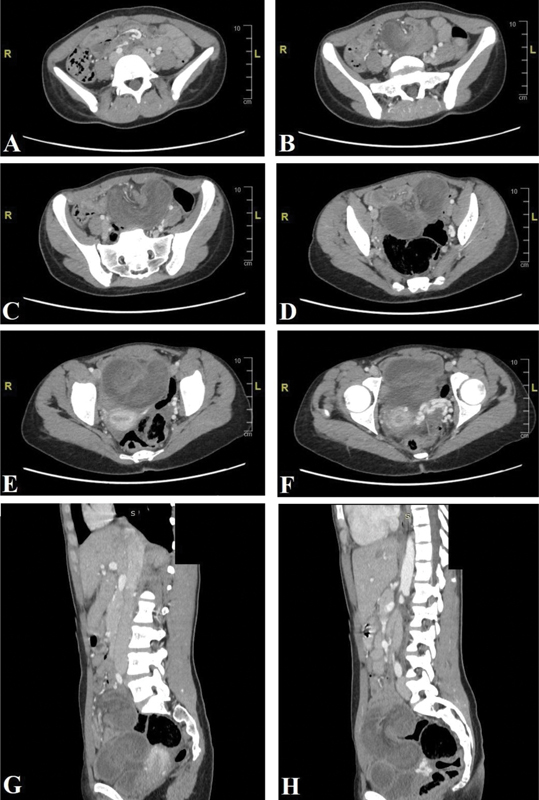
Computed tomography scan of the abdomen and pelvis + intravenous contrast.
